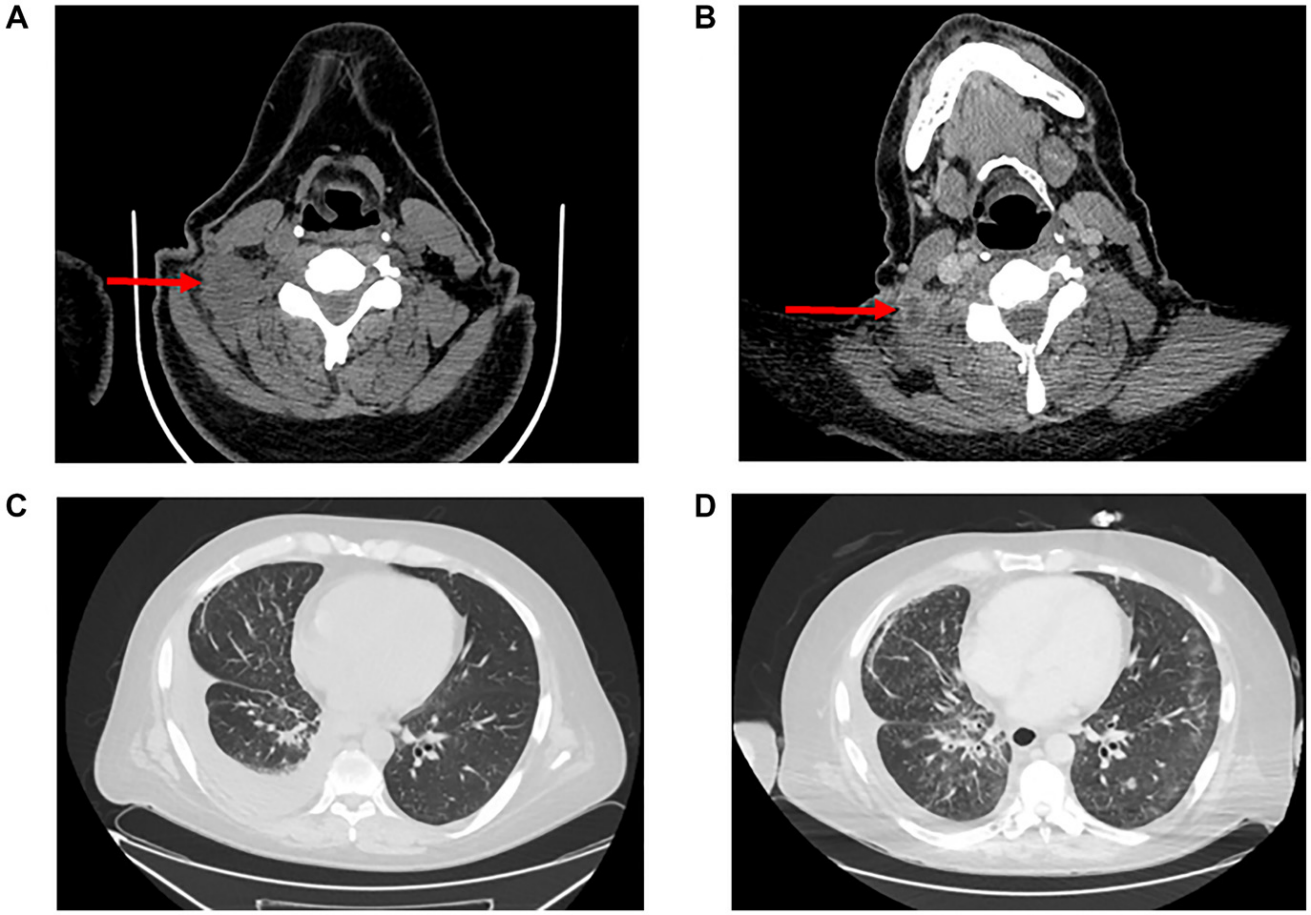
Radiographic response imaging to pralsetinib plus lorlatinib. (A) Computed tomography (CT) during lorlatinib treatment revealing right cervical lymph node. (B) CT showing decrease in lymph node size with necrosis after addition of pralsetinib. (C) Chest CT during lorlatinib treatment showing right-sided pleural effusion. (D) Chest CT showing decrease in effusion after pralsetinib treatment. Images A and C were taken on day 1135 post-diagnosis. Images B and D were taken on day 1268.
